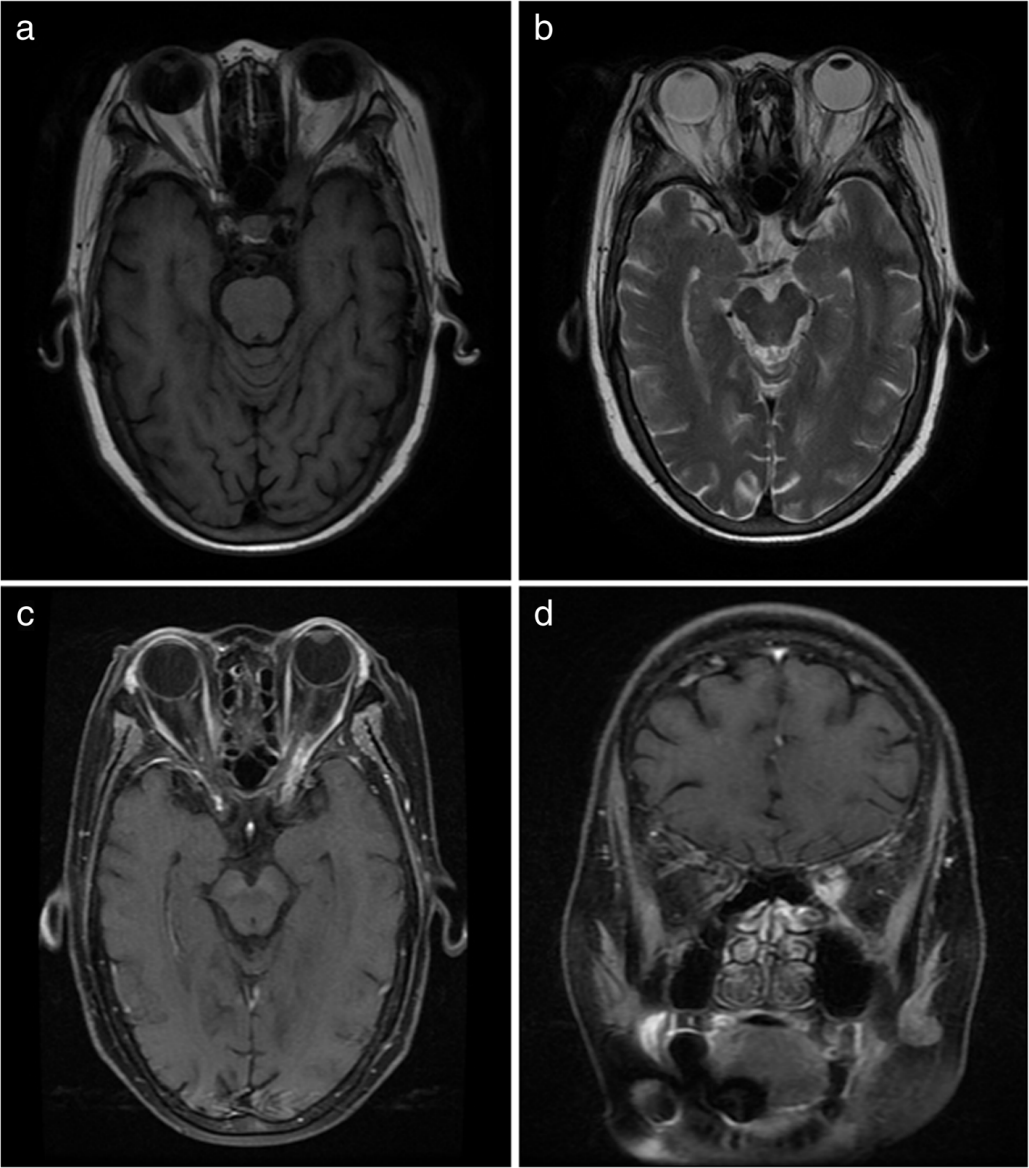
MRI presentations of mucormycosis presented with orbital apex syndrome. a: Axial T1WI MR shows a isointensity lesion in the left orbital apex;b: Axial T2WI MR shows a hypointensity lesion in the left orbital apex and high signal in the sphenoid sinus; c: Axial contrast-enhanced T1WI MR shows a enhancing lesion in the left orbital apex; d: Coronal contrast-enhanced T1WI MR shows a enhancing lesion in the left orbital apex
